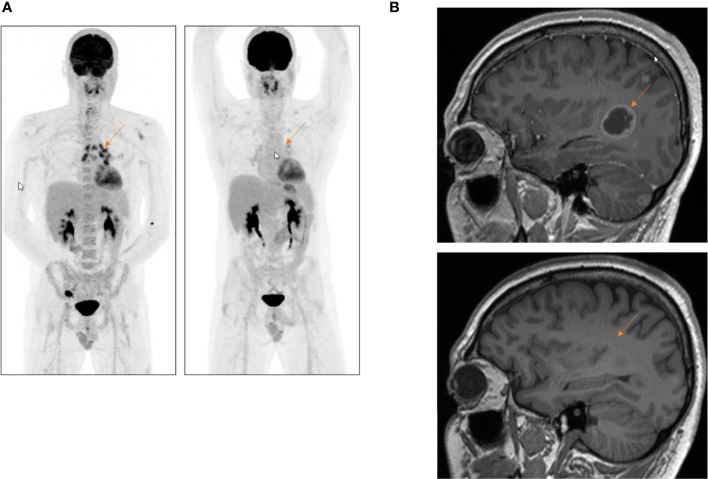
First-line treatment with alectinib in a 37-year-old patient with a metastatic *ALK*-rearranged LCNEC. (A) FDG-PET scan, whole-body overview, before (left) and 12 weeks after (right) initiation of first-line treatment with alectinib. (B) Complete response of the multiple brain metastases 10 weeks after the start of alectinib. Whole-brain radiation could be avoided. Red arrow shows excellent tumor response under treatment with alectinib.
